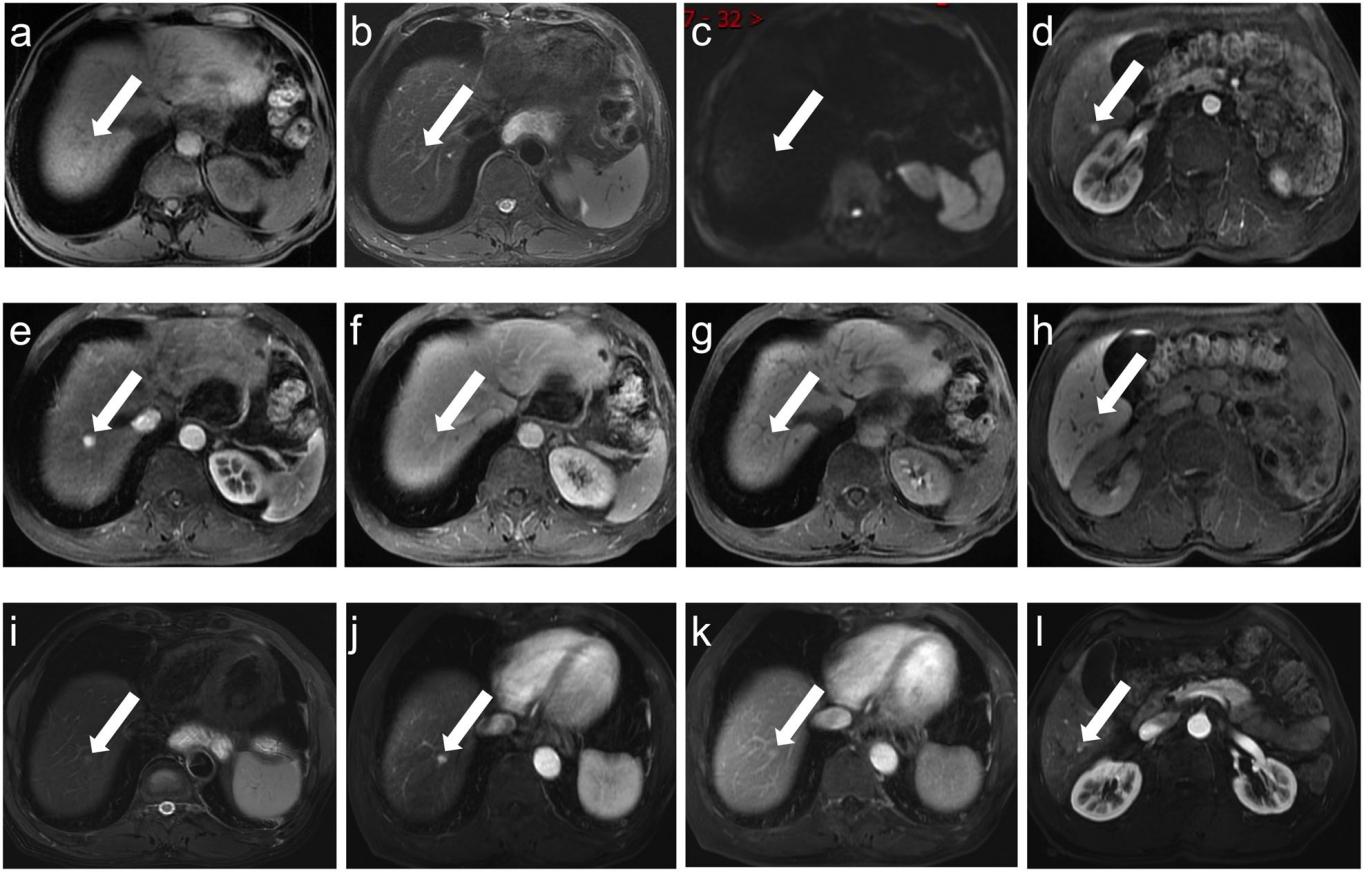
A 63-year-old male with gastric cancer treated with surgery and adjuvant FOLFOX chemotherapy regimen (10 cycles). At 29.7 months after completion of treatment, a nodule (white arrow) showed slightly hypointense on T1WI (a), slightly hyperintense on T2WI (b), isointense on DWI (c), hyperintense on AP image (e), without washout on DP image (f), and ring hyperintense on HBP image (g). Noteworthy, d (AP) and h (HBP) showed another hepatic FNH. After 49.0 months, T2WI (i), AP (j, l), and DP (k) images showed a decrease in the size of both nodules. AP, arterial phases; DP, delayed phases; HBP, hepatobiliary phases
